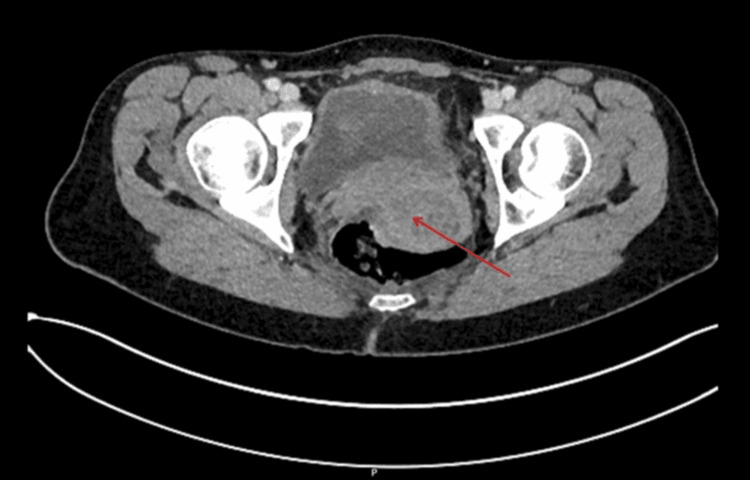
Computed tomography of the abdomen and pelvis showing bulky uterus (red arrow) with enlarged uterine cavity
